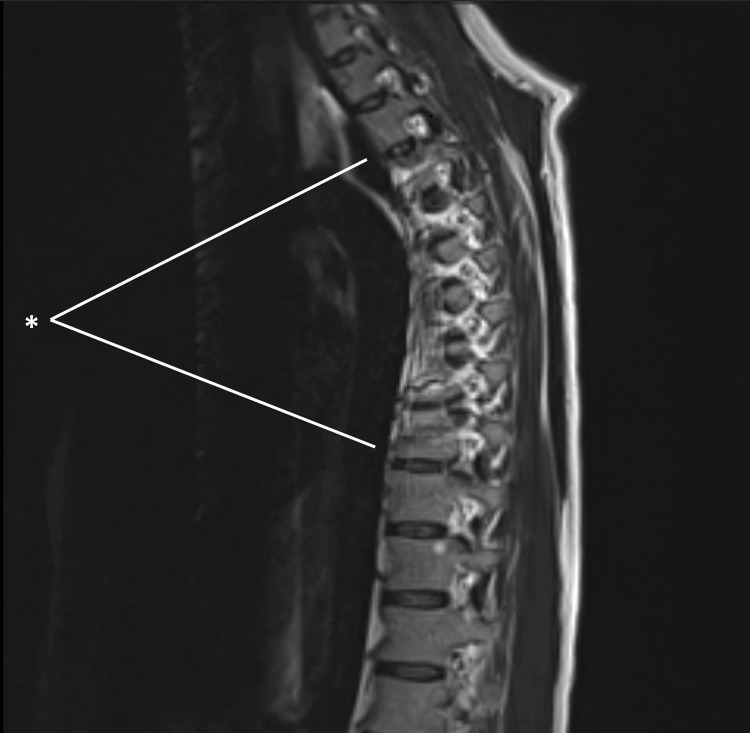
MRI demontrating osteomyelitis. Markers indicate T4-T10 osteomyelitis. The MRI demonstrates an irregular area of hyperintensity within the thoracic spinal cord extending from the T4 to T10 levels. The central canal remains patent, and no neural foraminal stenosis is identified.
